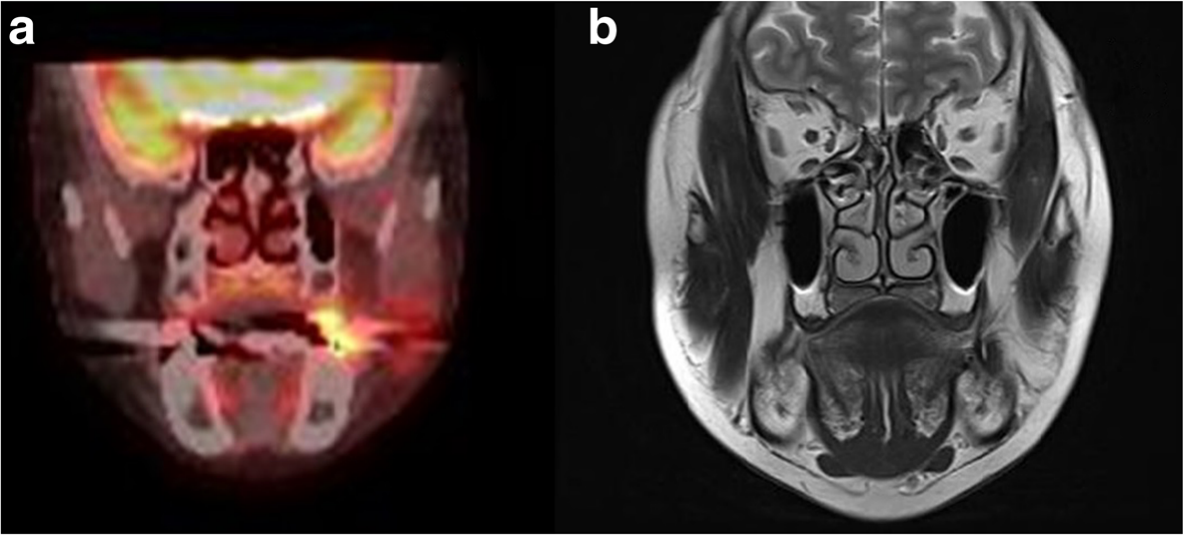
Radiological findings showing post-op state change on left buccal area. a 18-FDG PET/CT coronal view showing hot FDG spot on left buccal area because of post-operative change. b T2-weighted magnetic resonance image, coronal view
